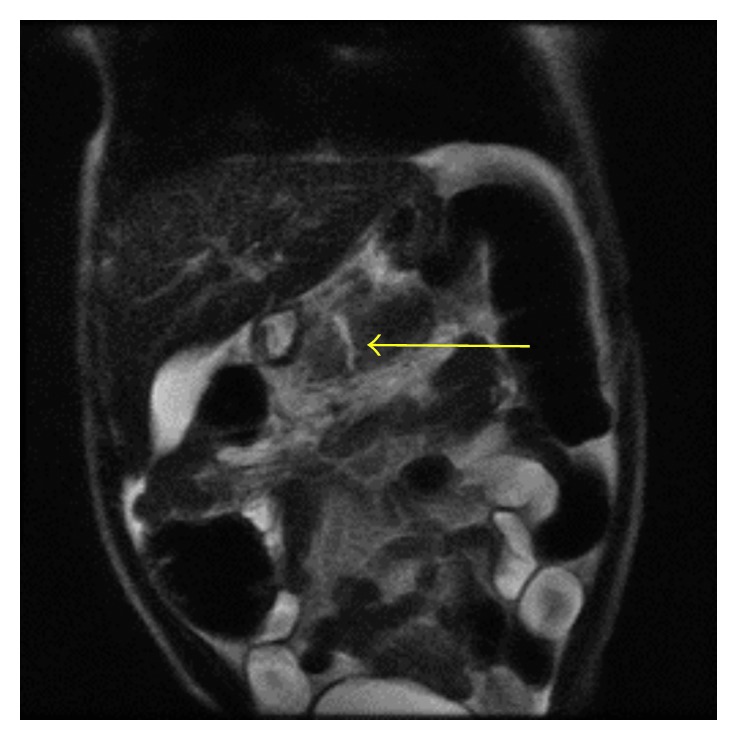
MRI showing complete transection through the proximal portion of the body of the pancreas.
